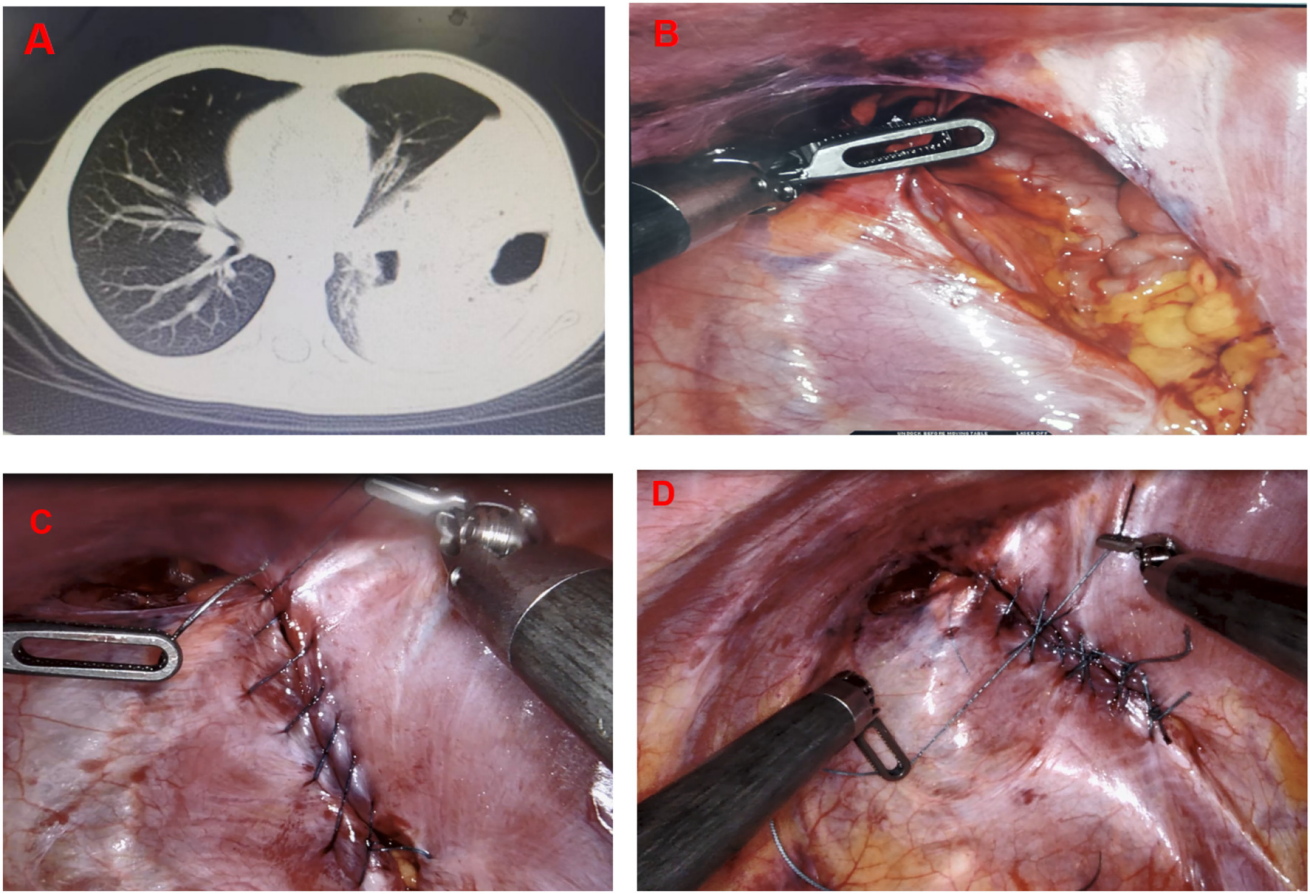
Surgical procedure of barbed suture combined with non-absorbable needle suture for thoracoscopic repair of congenital diaphragmatic hernia in children: (A) preoperative CT image showing herniation of abdominal contents into the thoracic cavity. (B) Intraoperative view revealing the diaphragmatic defect. (C) Continuous suturing of the defect using barbed suture to evenly reduce tension. (D) Reinforcement with interrupted non-absorbable needle sutures to ensure secure closure.
